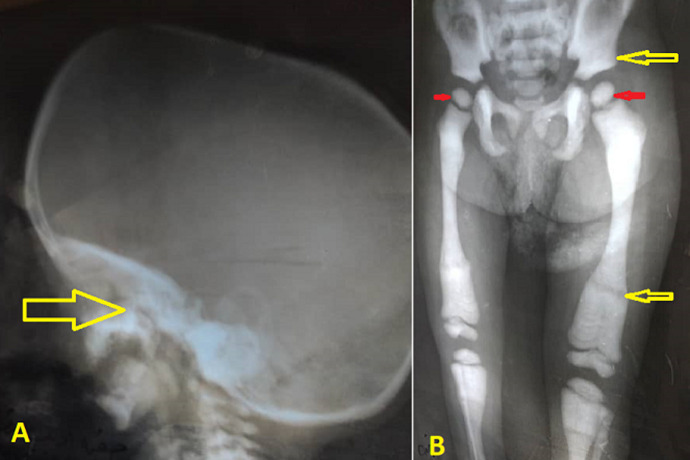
A) lateral skull radiograph showing increased thickness of skull base (arrow); B) increased bone density with little differentiation between cortex and medulla in long bone and pelvis (yellow arrows), metaphyseal modeling defects, and characteristic lucent bands (red arrow)
